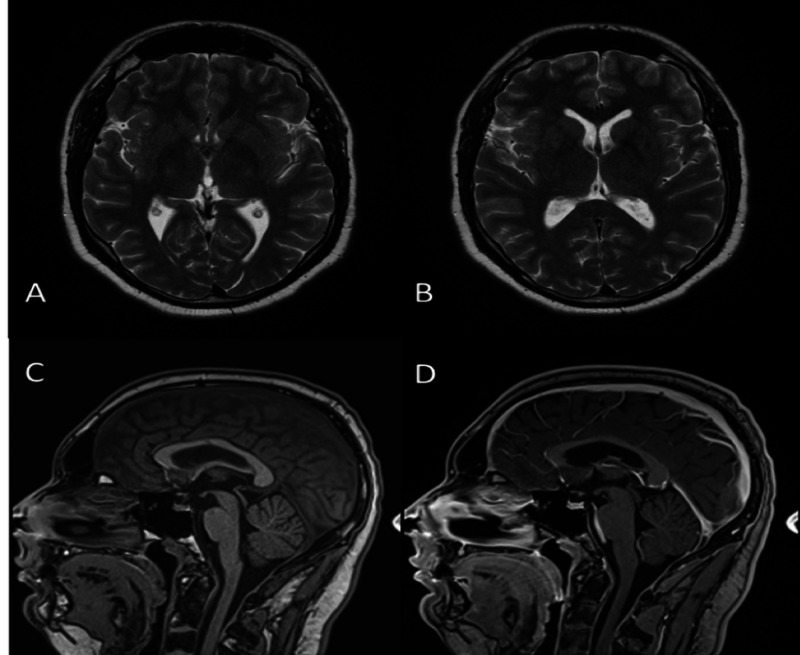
Post-treatment MRI of the brain. Axial T2-weighted MR image (A,B) shows interval resolution of the hydrocephalus post-ventriculostomy. Pre and post-contrast sagittal T1 weight MR image respectively (C, D) showing the absence of the pineal mass post-chemotherapy and radiotherapy. MRI, magnetic resonance imaging; MR, magnetic resonance
